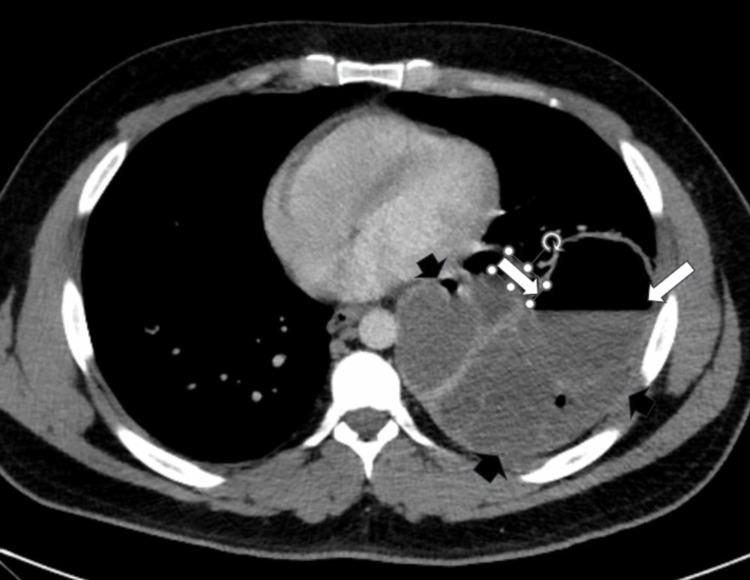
Admission chest CT scan Axial contrast-enhanced CT angiogram shows a large low attenuation lesion, which was measured as fluid density based on Hounsfield units with enhancing septations (short black arrows) with air-fluid level (long white arrows).
